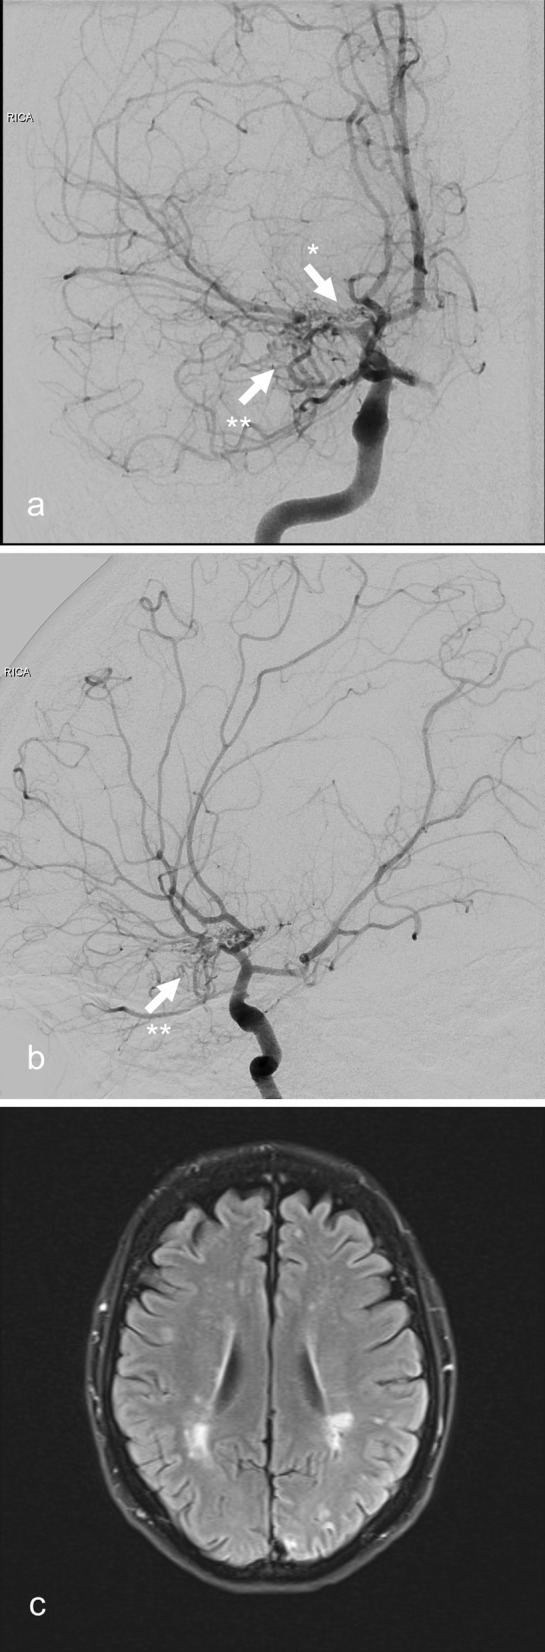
a Frontal and b lateral view of the catheter angiography of the index patient showing stenoses of the terminal right ICA and occlusion of the proximal right MCA (arrow with asterisk) as well as a Moyamoya-like basal collateral network (arrow with double asterisk); c MRI of the index patient’s mother showing white matter hyperintensities
